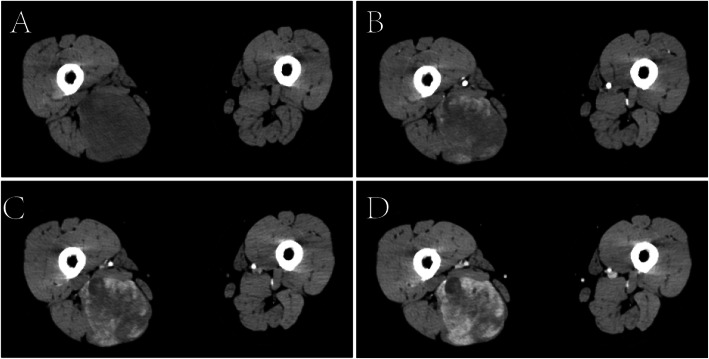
CT scan of right thigh showing a low-density mass with delayed enhancement. a plain scan, b arterial phase, c venous phase, d delayed phase
